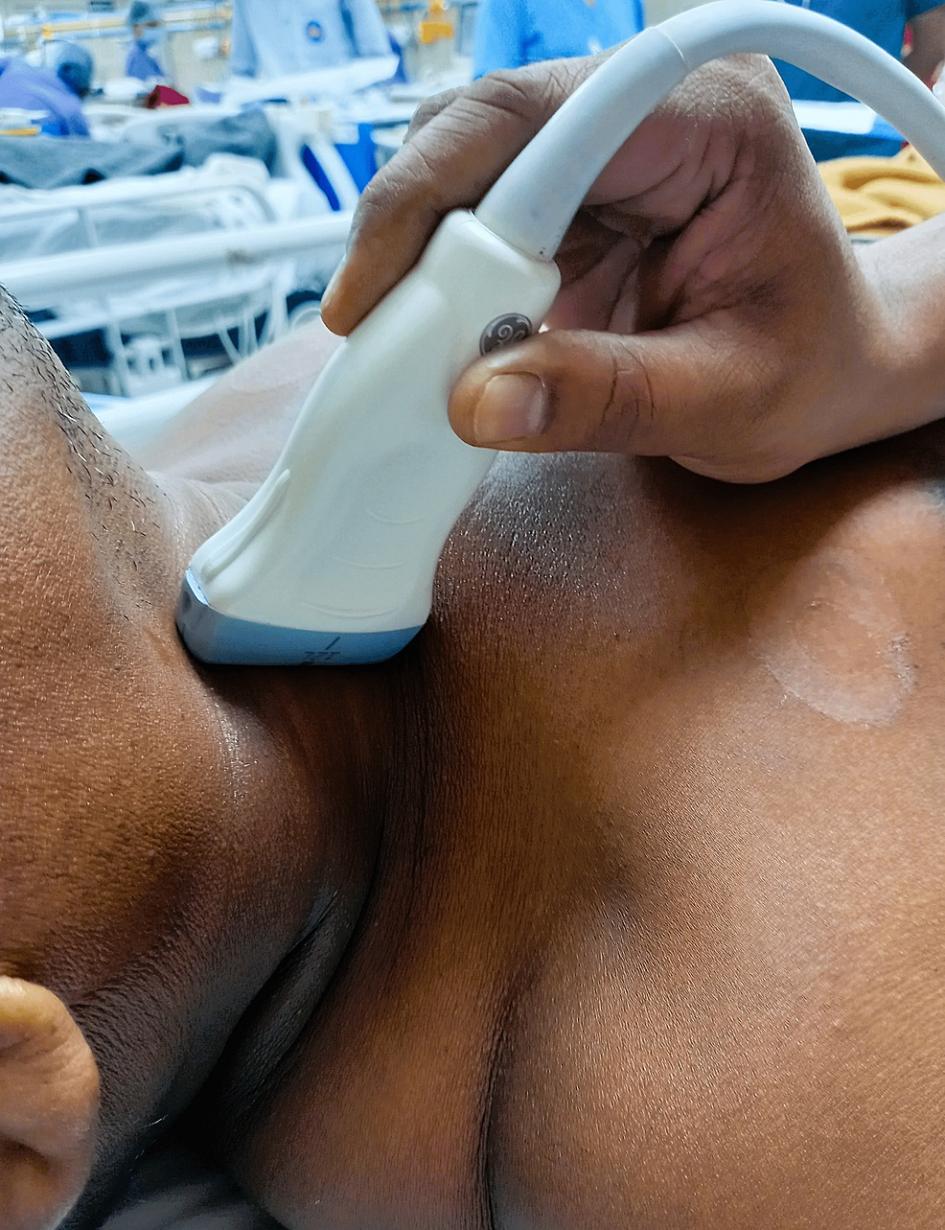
Probe position for carotid Doppler measurements (lateral view)
